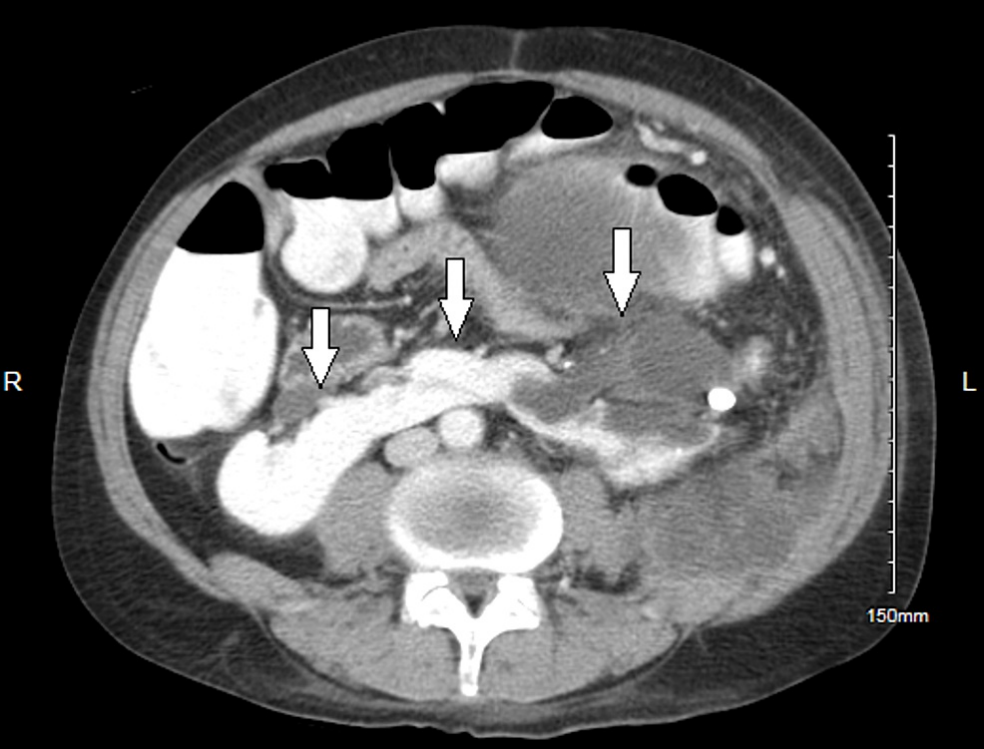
CT with contrast shows horseshoe kidney. CT: computed tomography.
